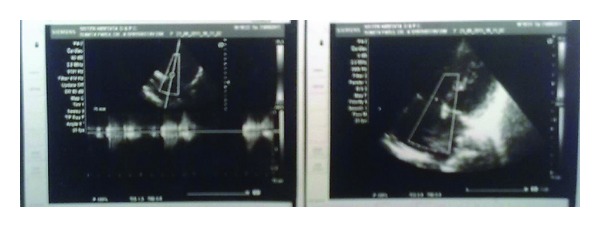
Echocardiographic view of ostium secundum atrial septal defect.
